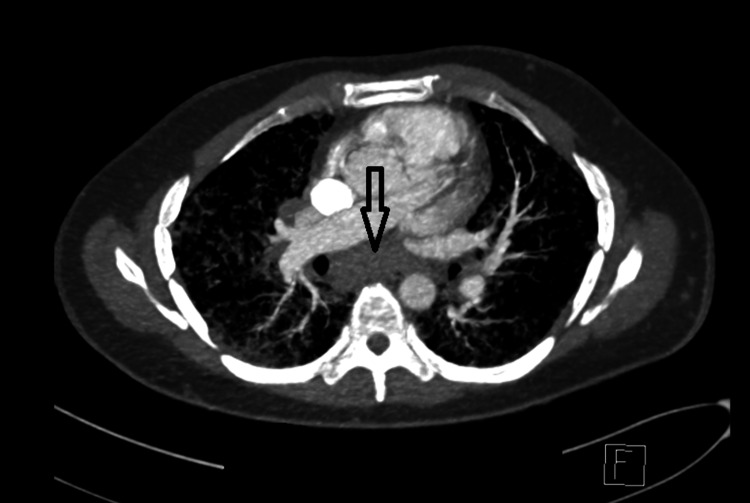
CT scan of the chest, with the arrow pointed to hilar lymphadenopathy.
